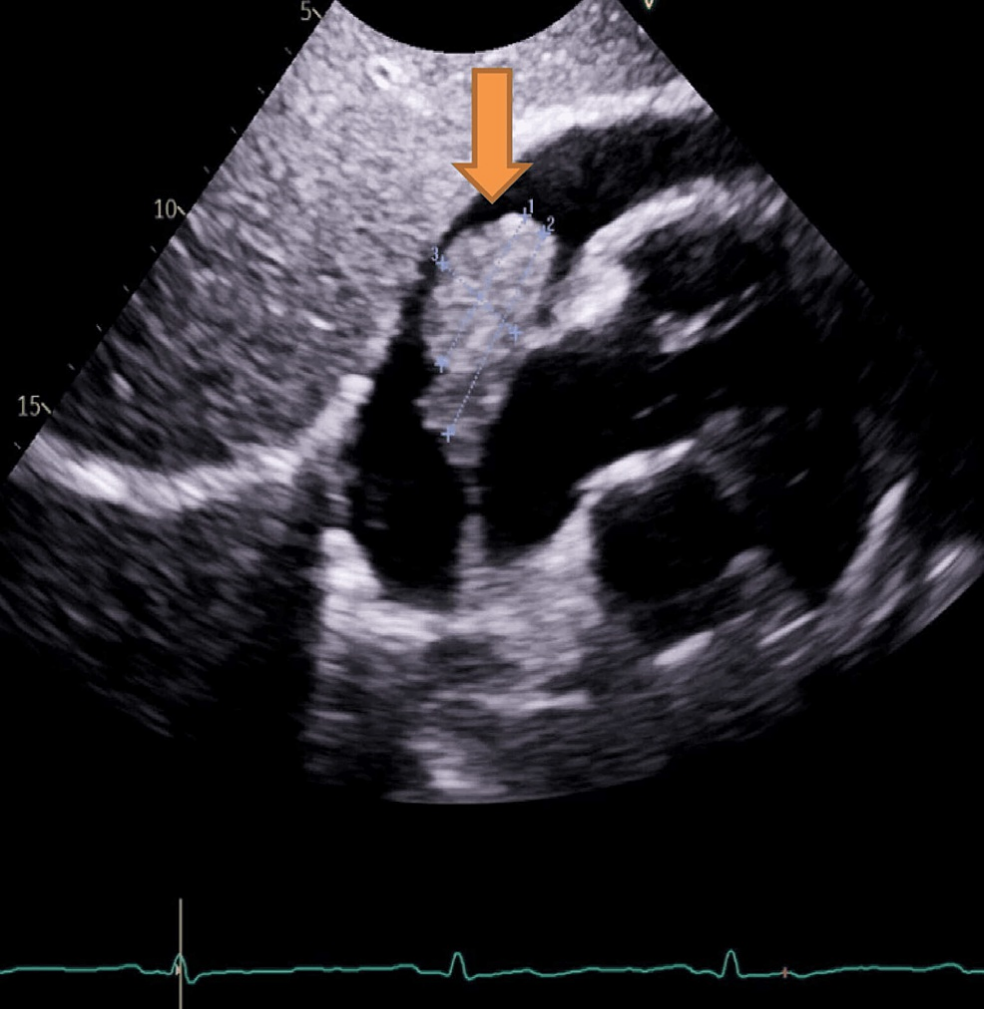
Echocardiography subcostal view showing large pericardial effusion and a right atrial mass (coloured arrow)
